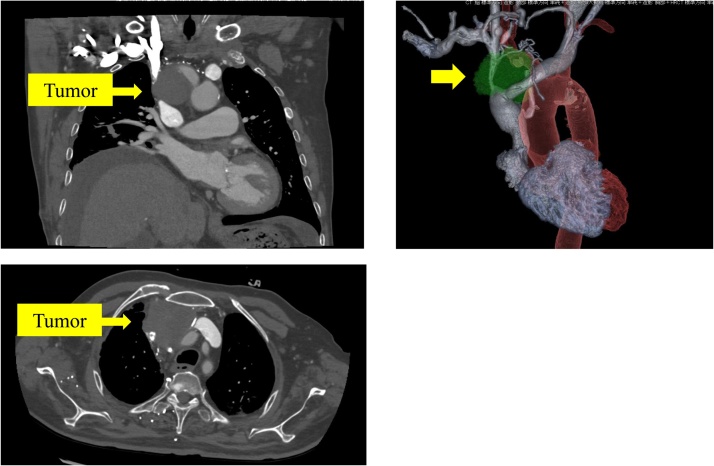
Chest computed tomography (CT) scan showing a 55 × 40 × 38-mm tumor located at the mediastinal lesion. This tumor had invaded the superior vena cava and both brachiocephalic veins.
